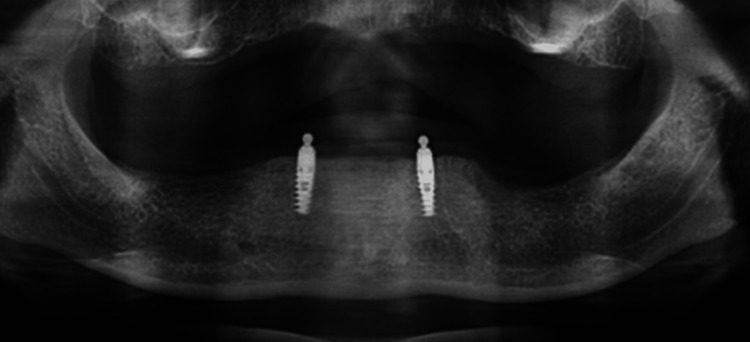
Radiograph after 6 years.
